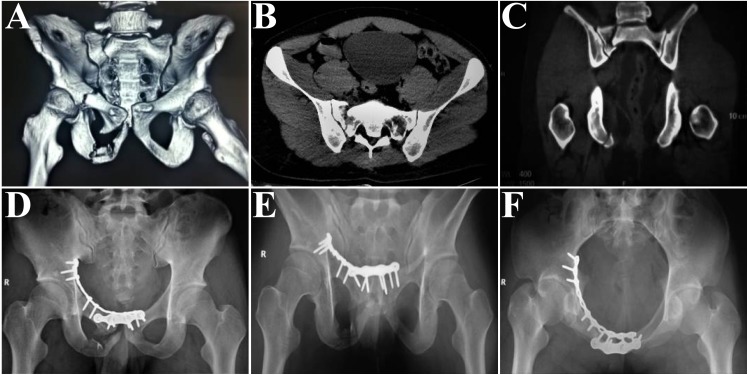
Representative case: a 27-year-old male patient was injured in a traffic crash. The anterior pelvic ring is treated surgically with the modified Stoppa approach and pelvic reconstruction plates for fixation of the anterior pelvic ring. The sacral fractures are treated conservatively. (A) Preoperative three-dimensional reconstruction image, showing unilateral superior and inferior pubic ramus fractures, with right complete, nondisplaced sacral Denis zone 2 fracture. (B) The complete, nondisplaced sacral fracture on an axial computed tomography (CT) scan. (C) The complete, nondisplaced sacral fracture on the coronal CT scan. (D) Postoperative anterior-posterior plain. (E) Postoperative outlet plain. (F) is the postoperative inlet plain.
